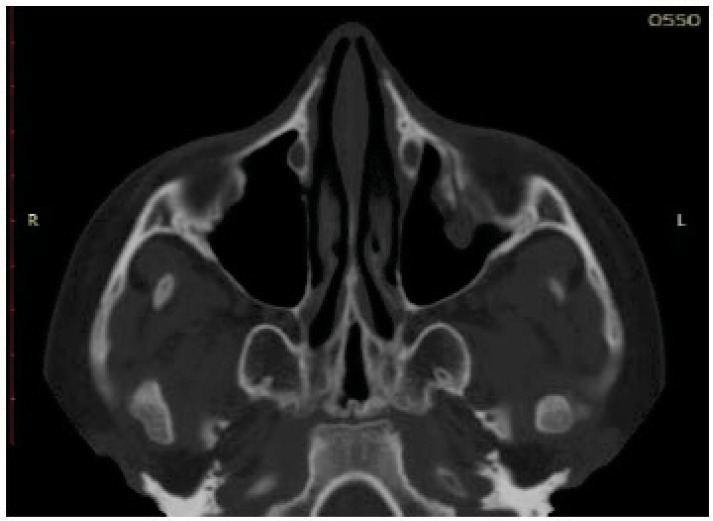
Preoperative CT axial projection. The left side of the sinus showed the presence of soft tissue inside.Preoperative CT axial projection. The left side of the sinus showed the presence of soft tissue inside.
